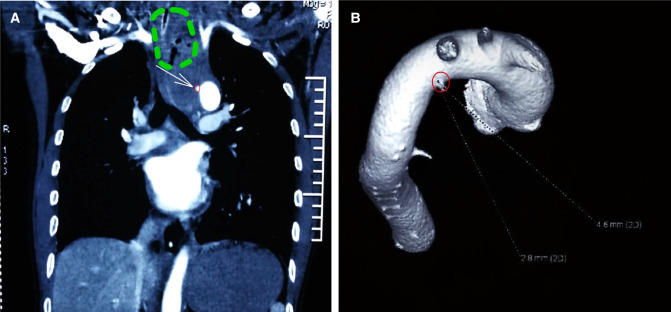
Preoperative examinations. (A) Computed tomography angiography (CTA): coronal view of mediastinitis and aortoesophageal fistula. Mediastinitis and gas accumulation with the green circle. The white arrow points to the leakage of contrast from the aorta, indicating a break in the aortic wall, within a small red circle. (B) Three-dimensional CT showed a break in the aortic wall (big red circle points to the aortic breakage).
